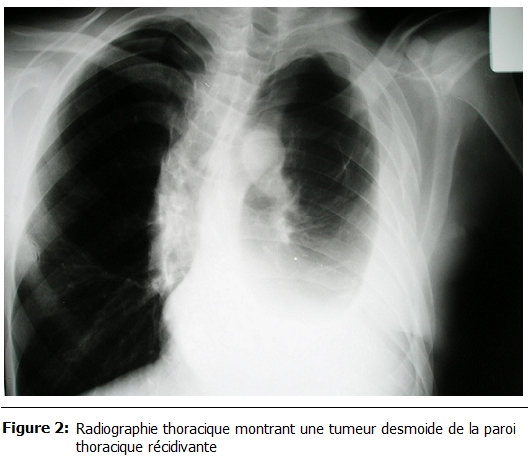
Radiographie thoracique montrant une tumeur desmoide de la paroi thoracique récidivante
